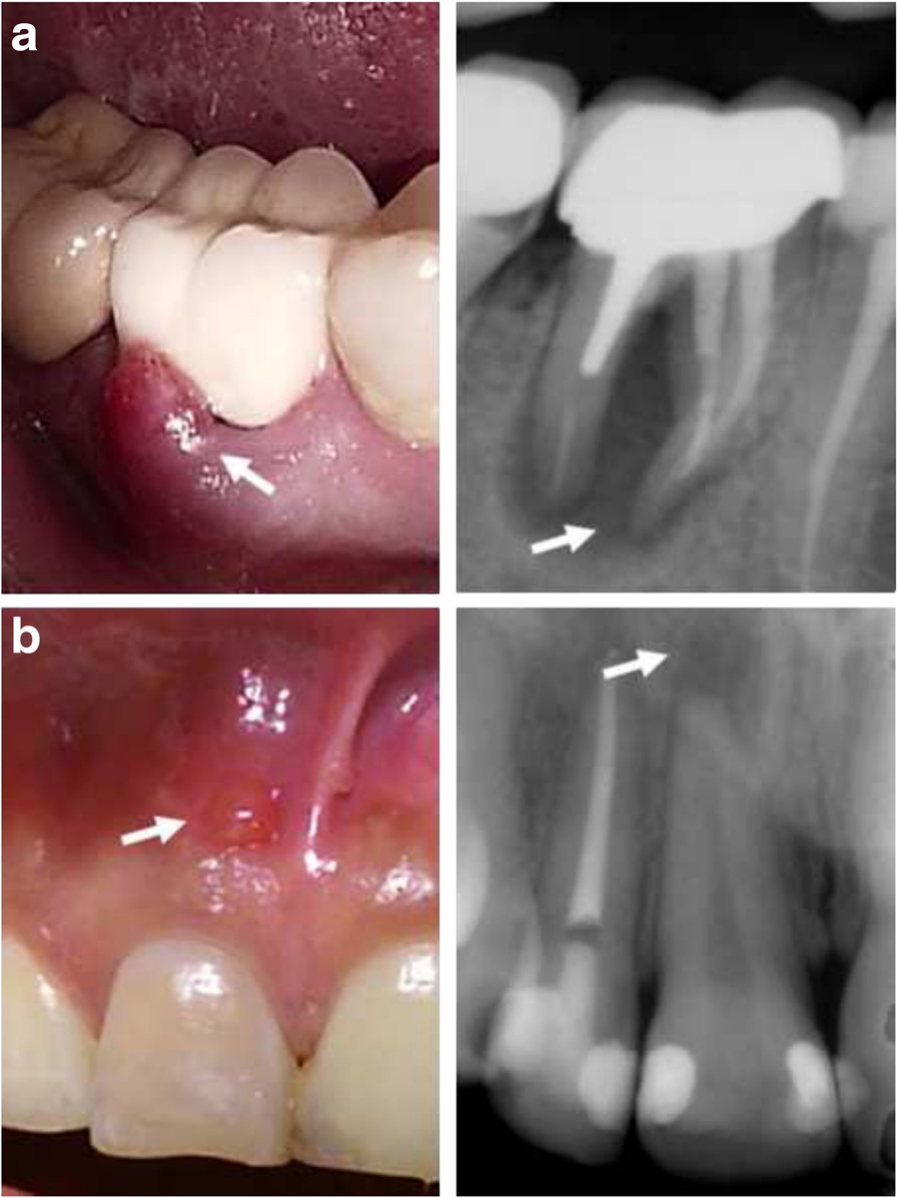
Clinical and radiographical presentation of mature teeth with chronic apical abscesses. a Non-restorable tooth with sinus tract and periapical radiolucency (*arrow*) excluded from the study. b Restorable tooth with sinus tract and periapical radiolucency (*arrow*) included in the trial
